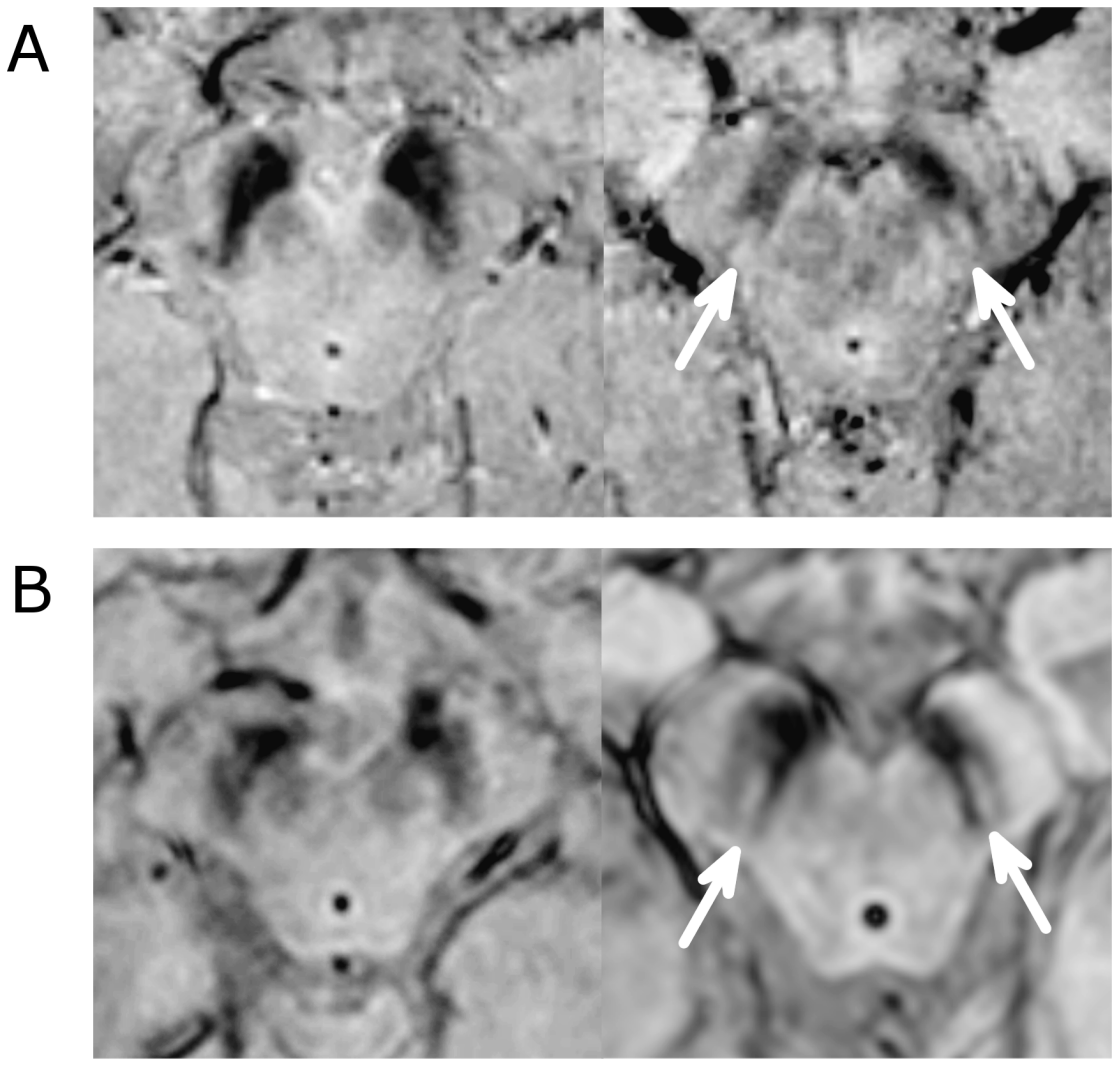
SWI MRI in PD and Non-PD patients. A. High resolution SWI MRI (3D gradient echo EPI, magnitude image) of a PD patient (left, 60 years, female, UPDRS: 53, HY score 3, nigrosome-1 absent bilaterally) and a control (right, 61 years, female, nigrosome-1 present bilaterally). B. Clinical high resolution 3D-T2*/SWI MRI (Philips ‘PRESTO’ sequence), of a PD patient (left, 58 years old, male, nigrosome-1 absent bilaterally) and a non-PD patient (right, 70 years old, female, diagnosed with an aneurysmal subarachnoid haemorrhage, nigrosome-1 present bilaterally).
